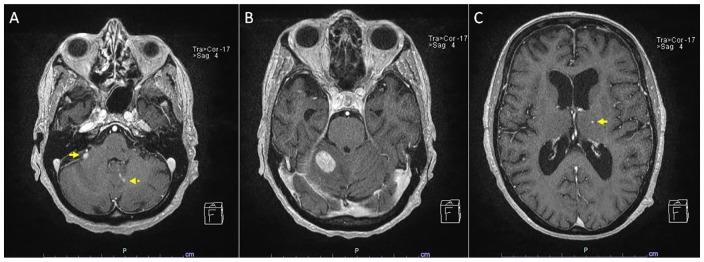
Brain MRI showing multiple enhancing brain lesions including a 0.7-cm right
